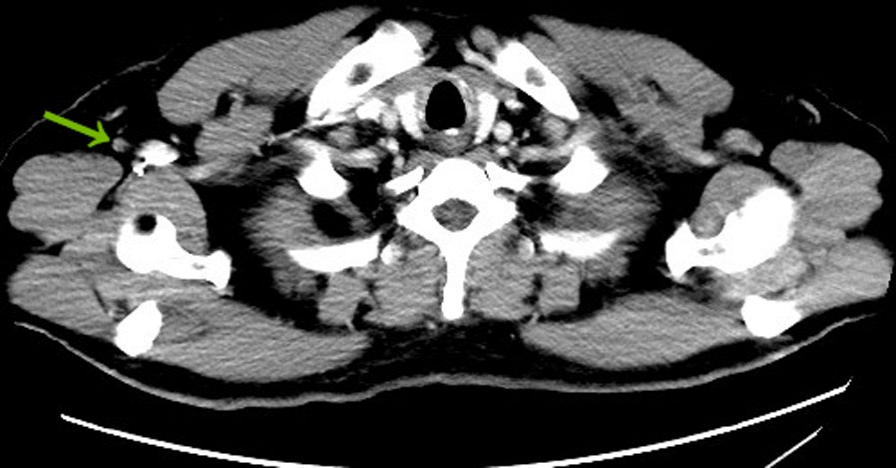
CT scan: postoperative changes of lymph nodes in the right axillary area (*arrow*). The density of soft tissue around this structure was increased
